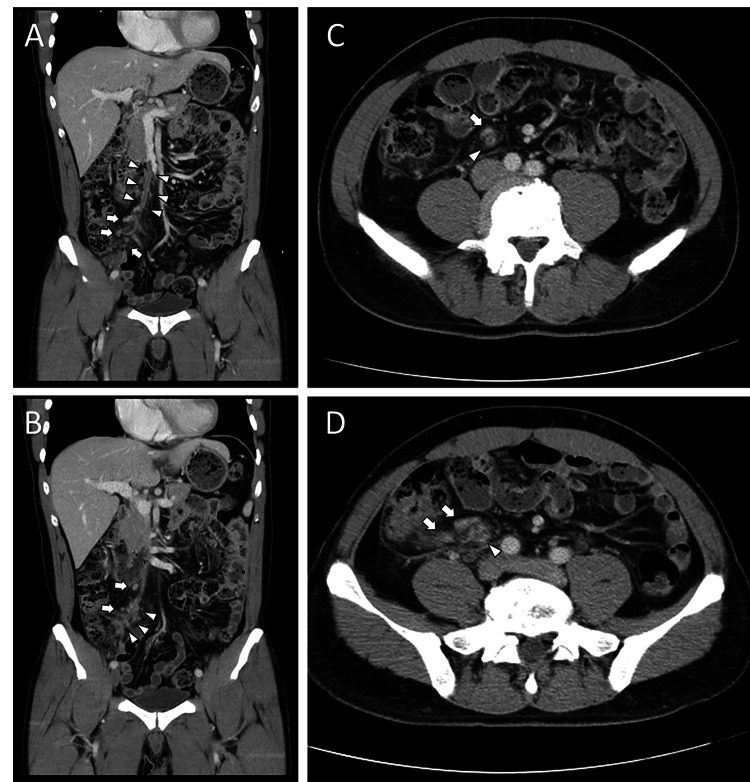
Contrast-enhanced abdominal CT scan Coronal plane (A and B) and axial plane (C and D) of contrast-enhanced CT revealed thrombus (white arrowheads) in the ileocolic branch of superior mesenteric vein with increased regional lymphadenopathy (white arrows) and fat strandings.
